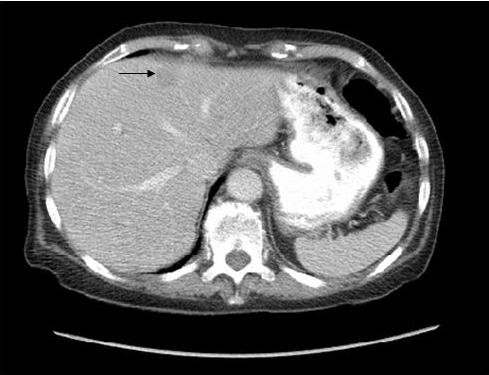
Abdominal CT scan showing liver lesion.
